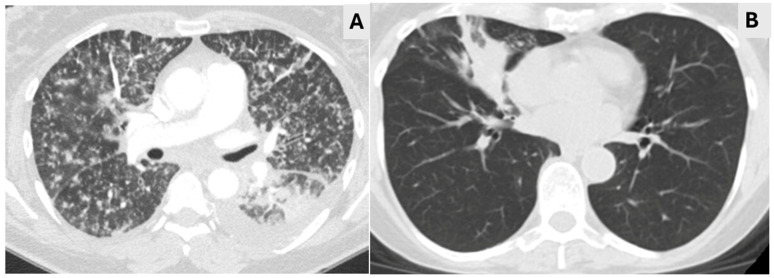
Acute pulmonary coccidioidomycosis. (A). CT of the thorax showing diffuse reticulonodular infiltrates in a woman who required intubation and mechanical ventilation. (B). CT of the thorax showing consolidated infiltrate in the right lower lobe.
